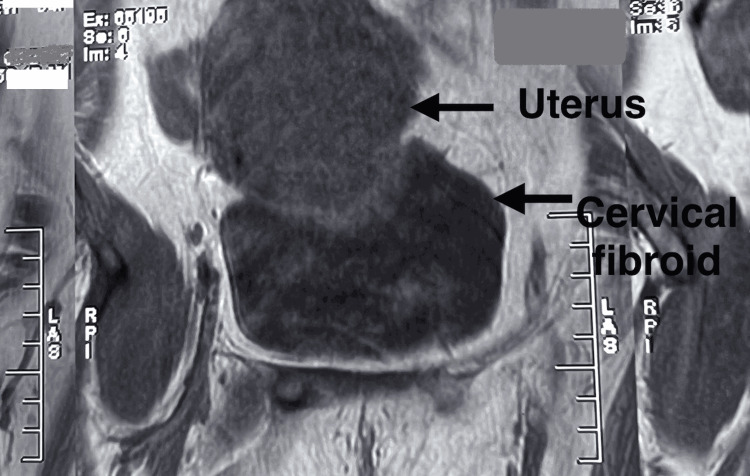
MRI revealed a uterus measuring 6.3 x 7.6 cm, with a large heterogeneous mass measuring 10 x 10 cm located at the lower uterine segment, suggestive of a cervical fibroid
